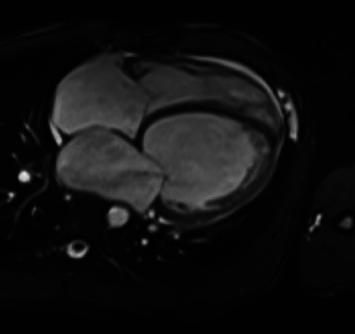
Cardiac magnetic resonance imaging showing a four-chamber view with evidence of biventricular dilatation.
